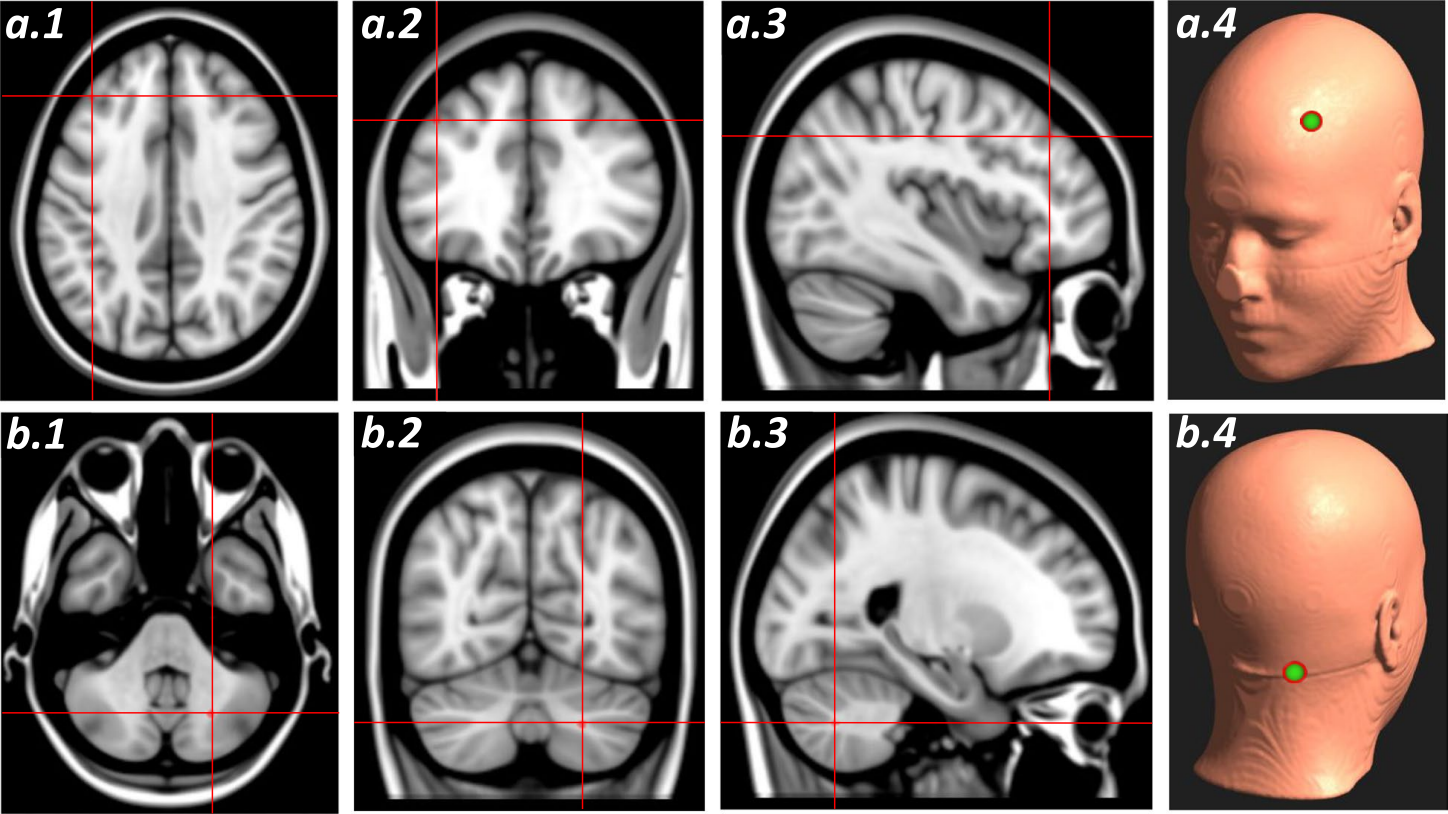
Transcranial tDCS targeted regions (used for electrode optimization and subsequent stimulation) assuming in the image a left hemisphere stroke. Established MNI (Montreal Neurological Institute) coordinates have been selected ensuring a constant electric field impact (0.25 mA) in Brodmann area 46 for the dorsolateral prefrontal cortex (DLPFC) and lobules  I–IV of theanterior cerebellar lobe (CEREB). A DLPFC target area (MNI coordinates *X* =  − 39, *Y* = 34, *Z* = 37) in an axial (*a.1*), coronal (*a.2*) sagittal (*a.3*) MRI sections and their scalp projection (*a.4*) views. B Cerebellar target area (MNI coordinates: *X* =  24, *Y* =  − 66, *Z* = 40) in axial (*b.1*), coronal (*b.2*), sagittal (*b.3*) MRI sections and their scalp projection (*b.4*) views. Target areas are represented in the MNI152 and labelled on a standard MRI model by means of an MRI-based frameless stereotaxic neuronavigation system (Brainsight)
